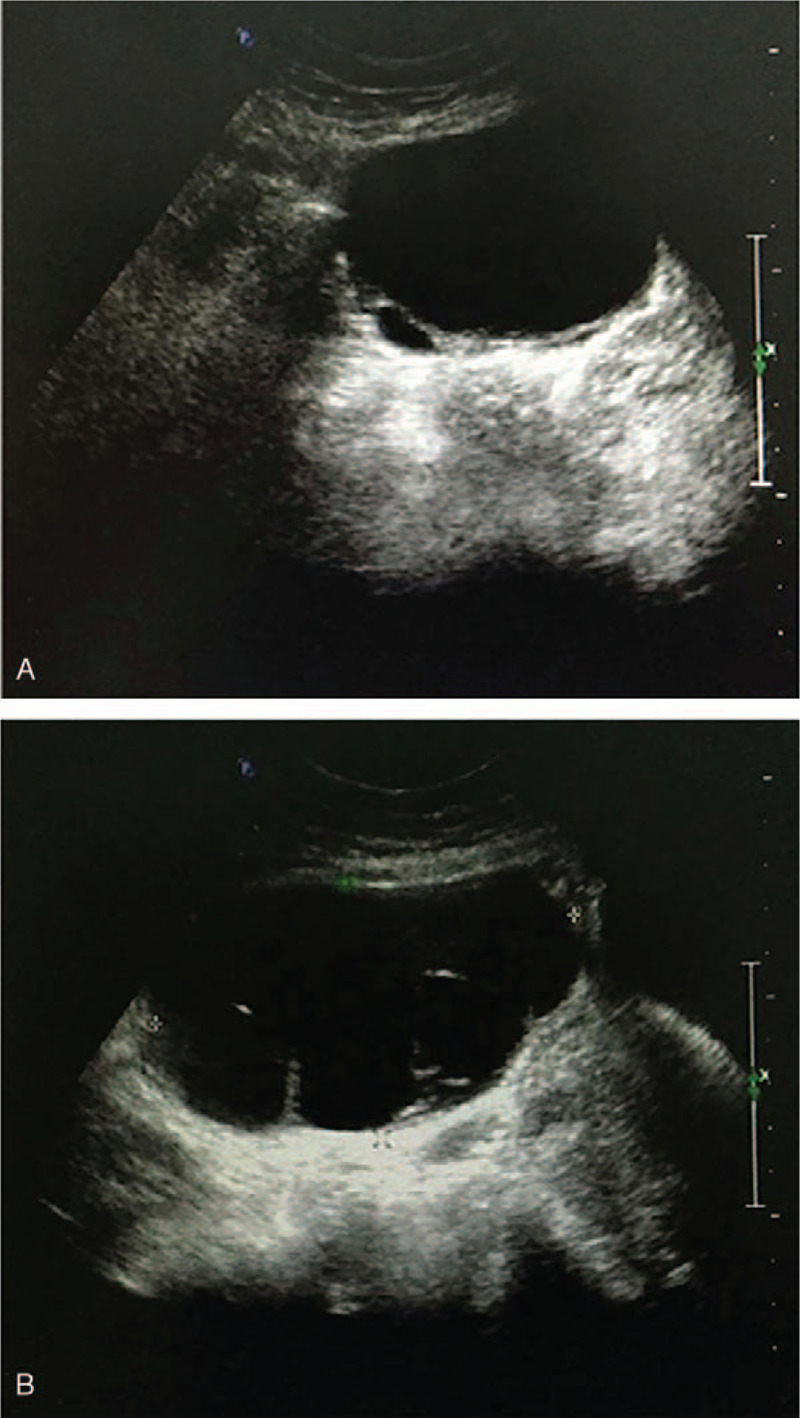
Ultrasonography revealed the completely anechoeic lesions of both ovaries suggestive of a purely cystic consistency. Please note that there were septum in the lesions. (A) Cystic lesion in the left ovary; (B) cystic lesion in the right ovary.
